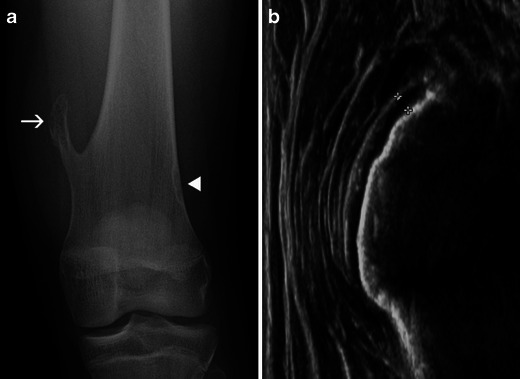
Pedunculated exostosis: a Frontal X-ray of the left knee demonstrates the typical features of exostosis (*arrow*); also note the cortical defect on the lateral femoral metaphysis (*arrowhead*). b Ultrasound measurement of the hypointense cartilage cap (*between calipers*)
